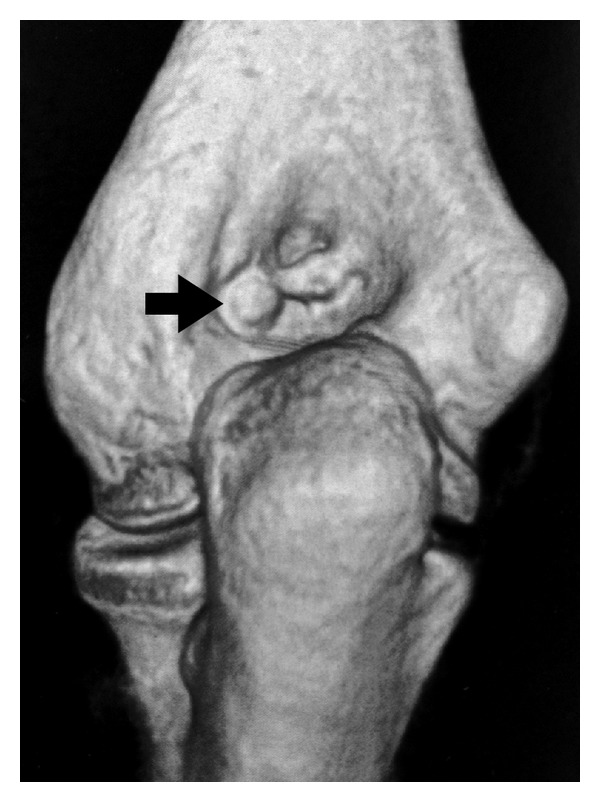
CT image reconstruction of the elbow: protrusion of a nidus in the lateral side of the olecranon fossa.
